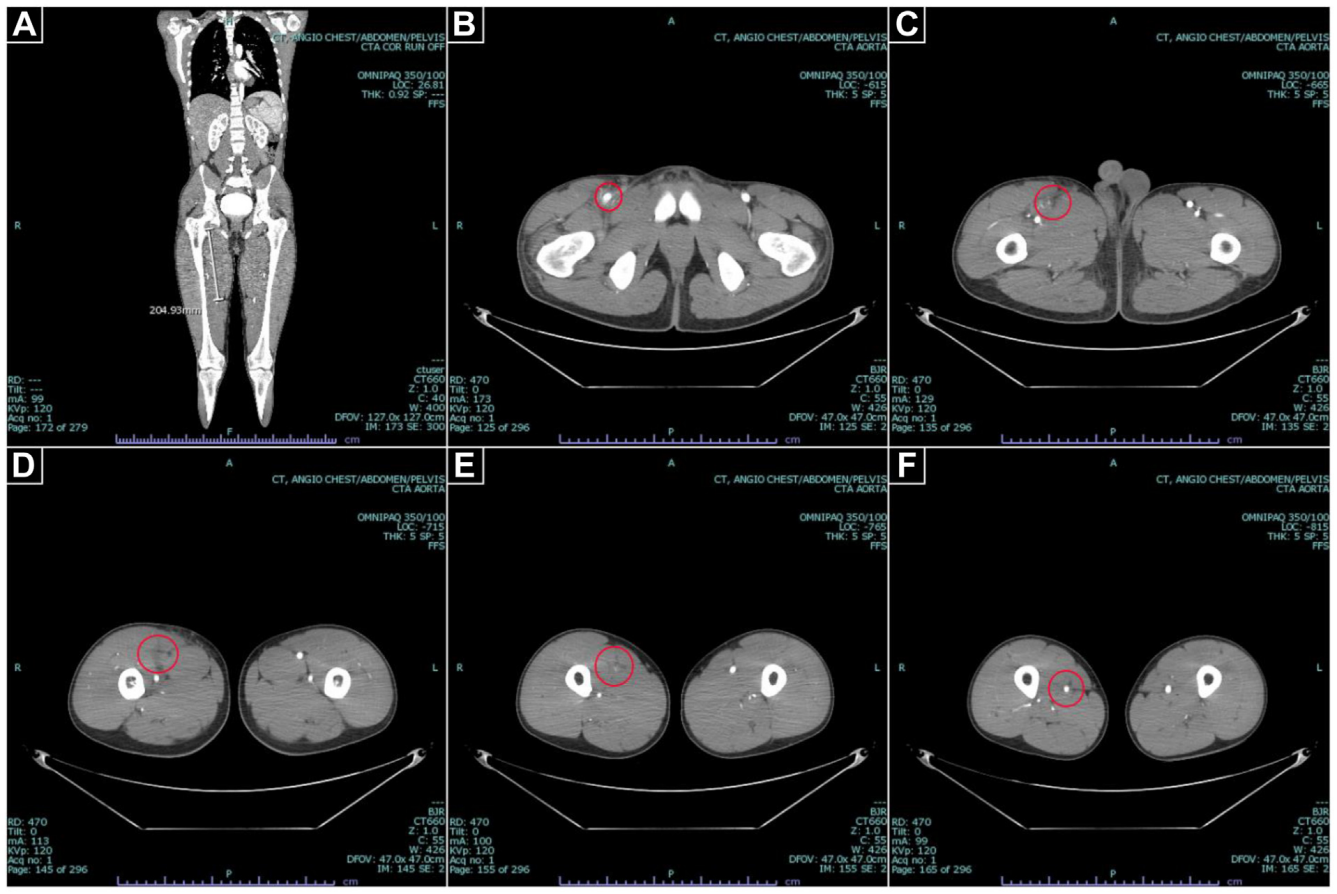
Computed tomography angiography (CTA) of the aorta with runoff. Representative CTA images demonstrate loss of arterial enhancement for an approximately 20-cm segment (A) of the right superficial femoral artery from its branch point with the femoris profunda (B) and reconstitution distally (F) with notable periarterial inflammation of the occluded segment (C-E).
